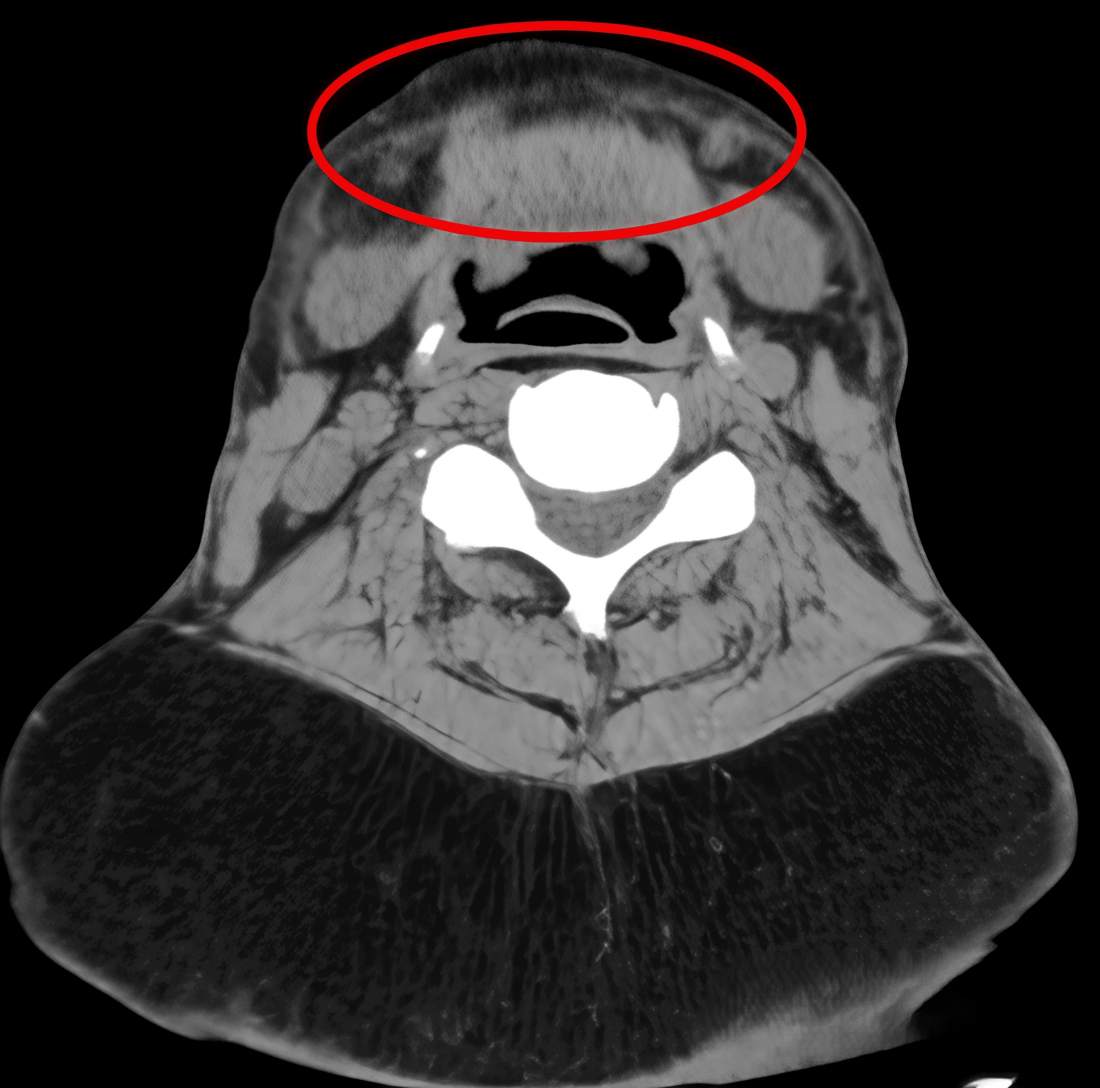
Postoperative CT imaging demonstrating platysmal anatomy Axial CT at the submental level showing midline platysmal diastasis (circled), contributing to residual soft-tissue laxity and neck contour irregularity.
